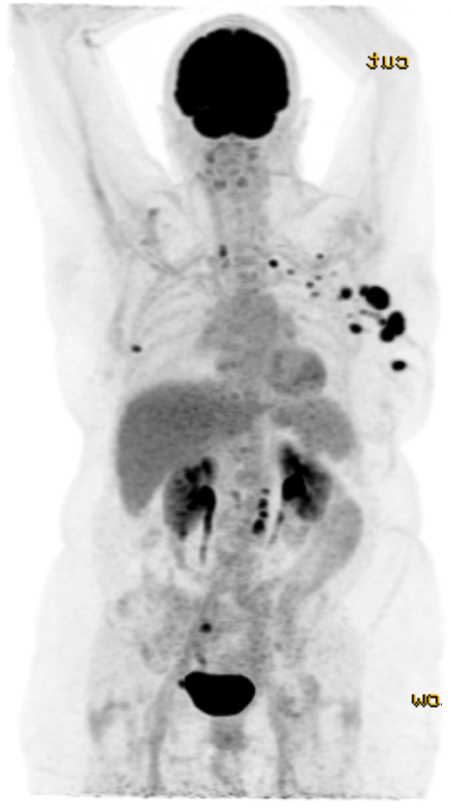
FDG PET CT demonstrating intensely FDG avid right-sided breast lesion with further intensely metabolically active left-sided axillary, internal mammary and mediastinal lymph nodes. FDG, fludeoxyglucose; PET, positron emission tomography.
